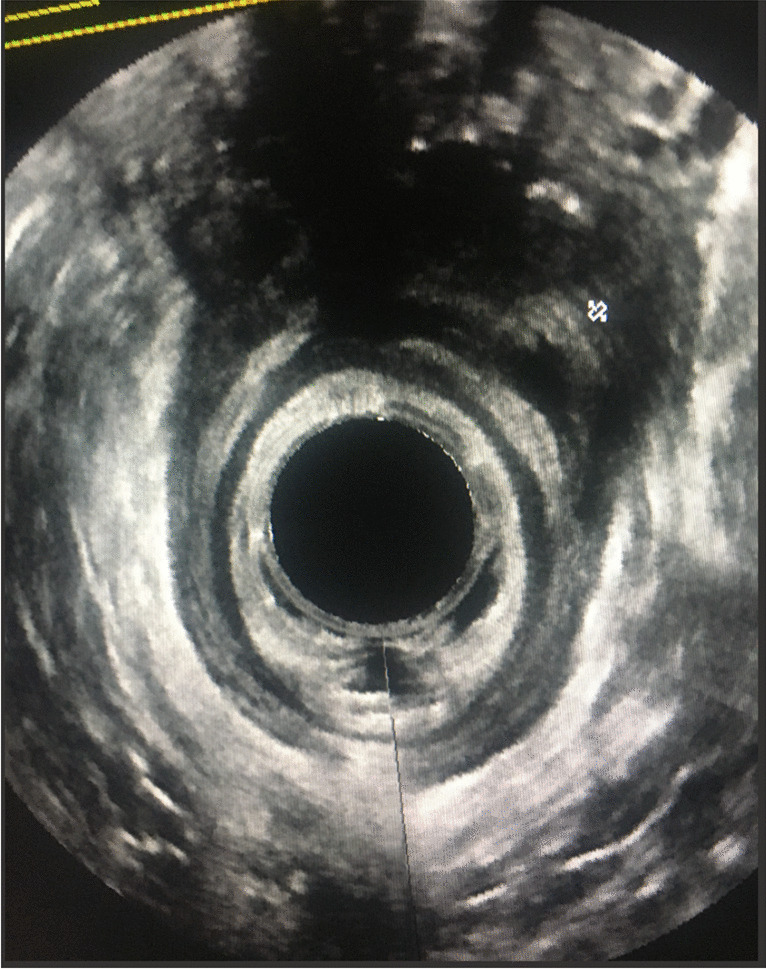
Endoanal ultrasound with internal and external anal sphincter defect
